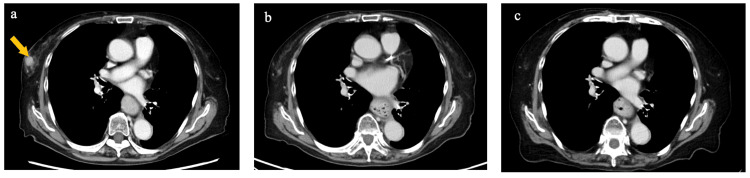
Computed tomography images of breast metastasis At nine months after the initial surgery (a), following eight courses (b), and following 27 courses of pembrolizumab treatment (c). After nine months, the tumor is barely detectable
